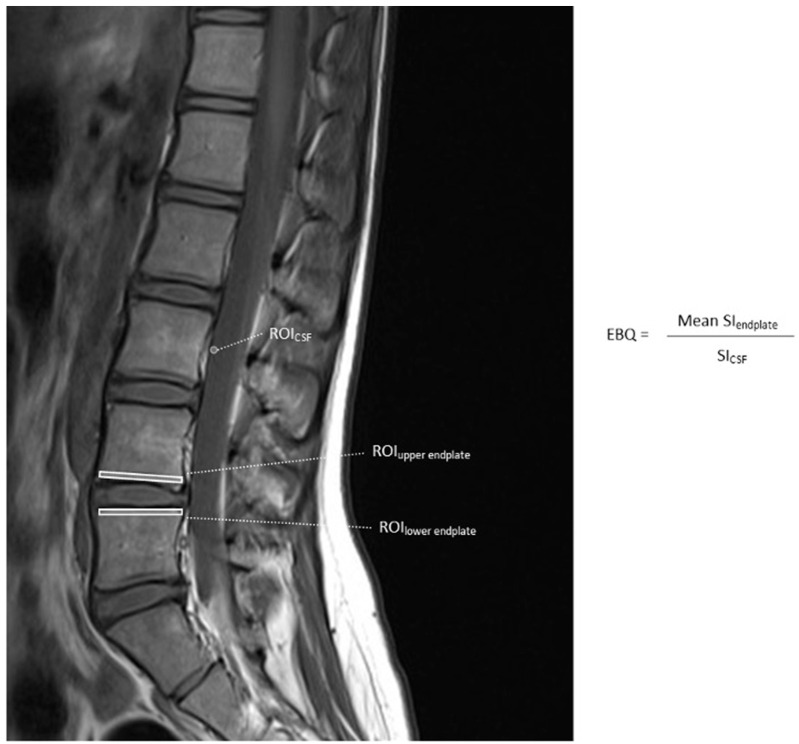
Non-contrast-enhanced T1-weighted MRI of the lumbar spine showing ROIs used to calculate L1–L4 vertebral bone quality (EBQ) score.
